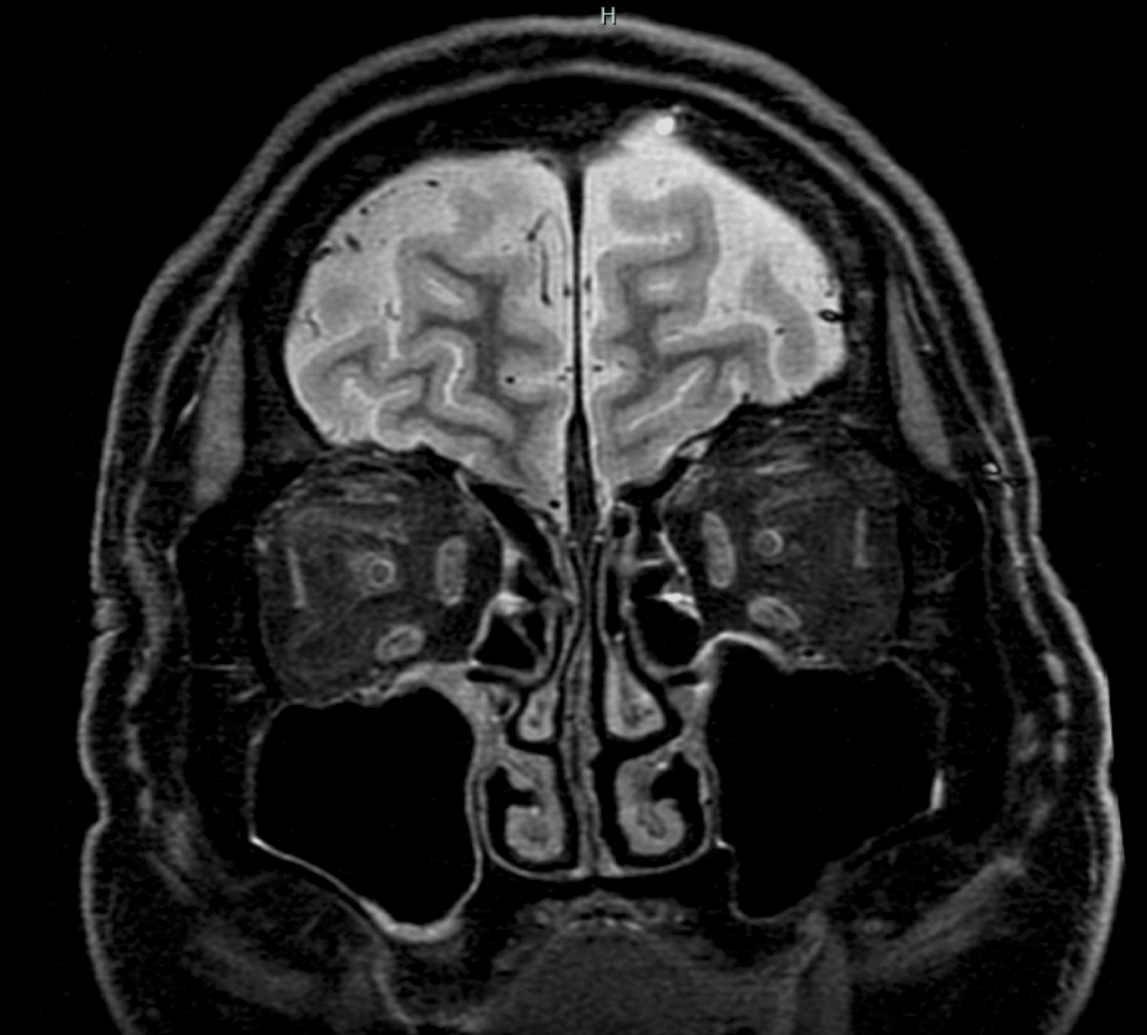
Brain MRI, non-contrast T1-weighted imaging, showed no significant abnormalities, such as optic nerve signal hyperintensity.
